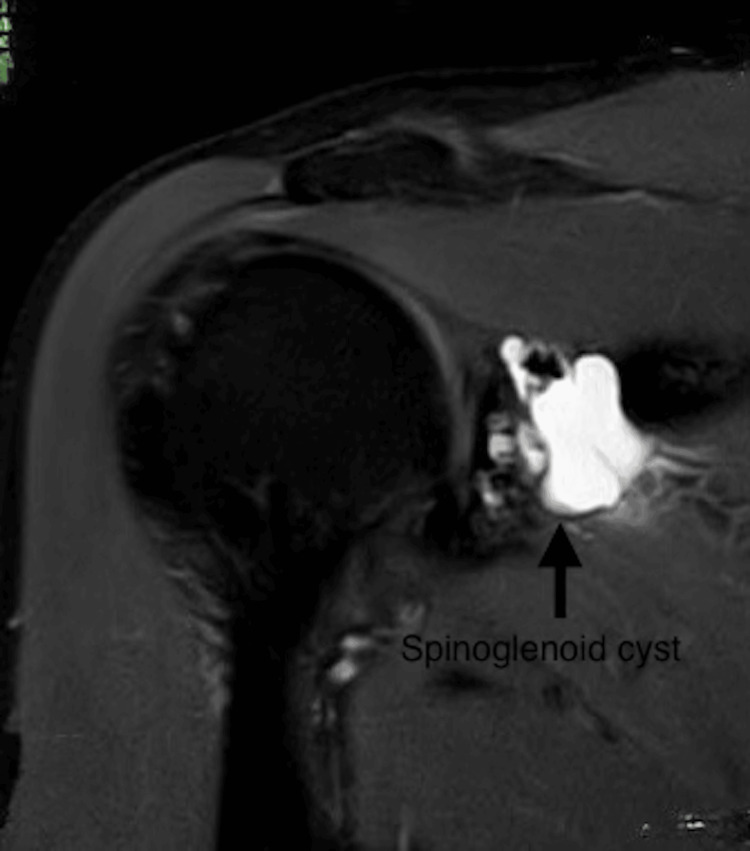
MRI of the right shoulder showing cystic lesion at spinoglenoid notch
